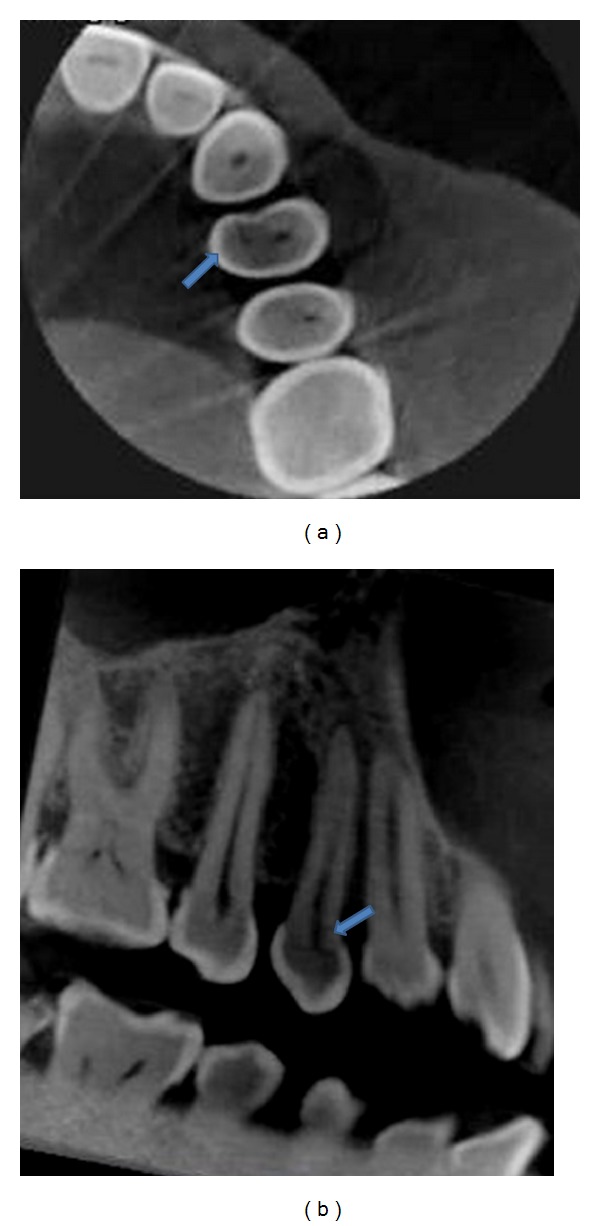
(a) Axial view of CBCT reveals a fracture line extending mesiodistally involving lingual pulp horn. (b) Sagittal view of CBCT reveals a fracture line extending mesiodistally at the level of pulp horn.
